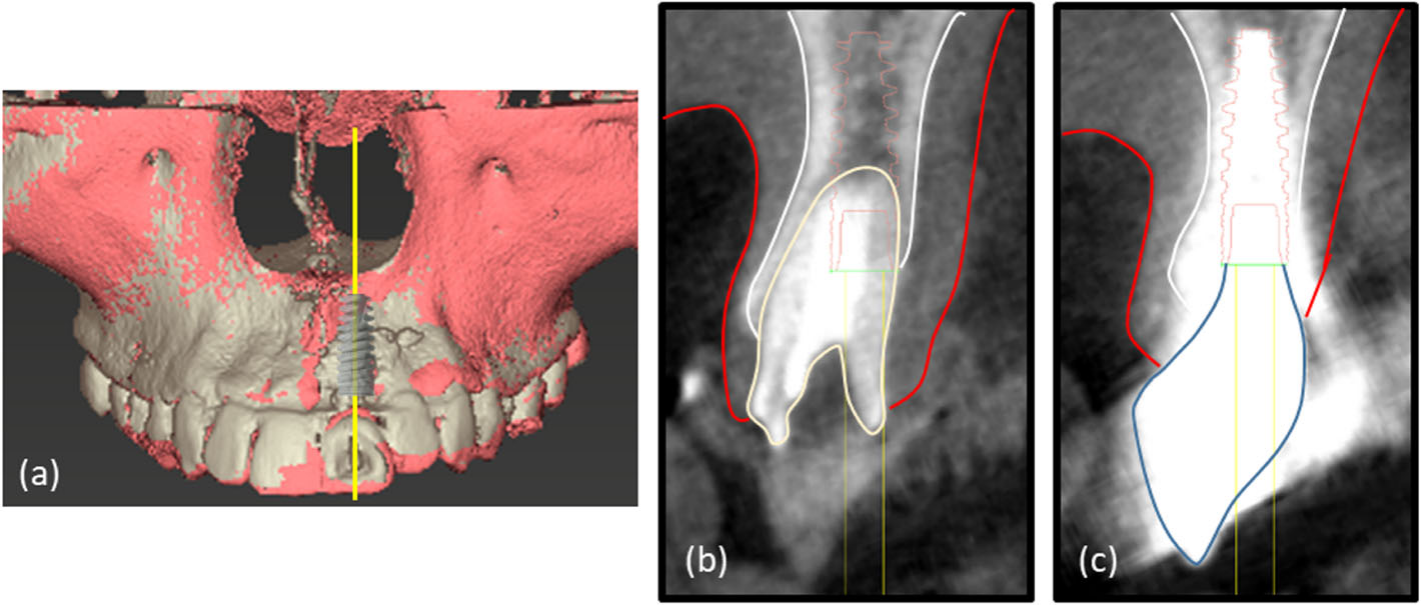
CBCT image acquisition preoperatively (T0) and 1 year after connection of the definitive prosthesis (T1). a Superimposition of 3D maxillary models obtained from cone-beam computed tomography scans at T0 and T1. b Buccopalatal cross-sectional images at T0 acquired with reference to the long axis of a virtual implant placed in the exact position where the actual implant would be. c Buccopalatal cross-sectional images at T1 acquired with reference to the long axis of the implant
